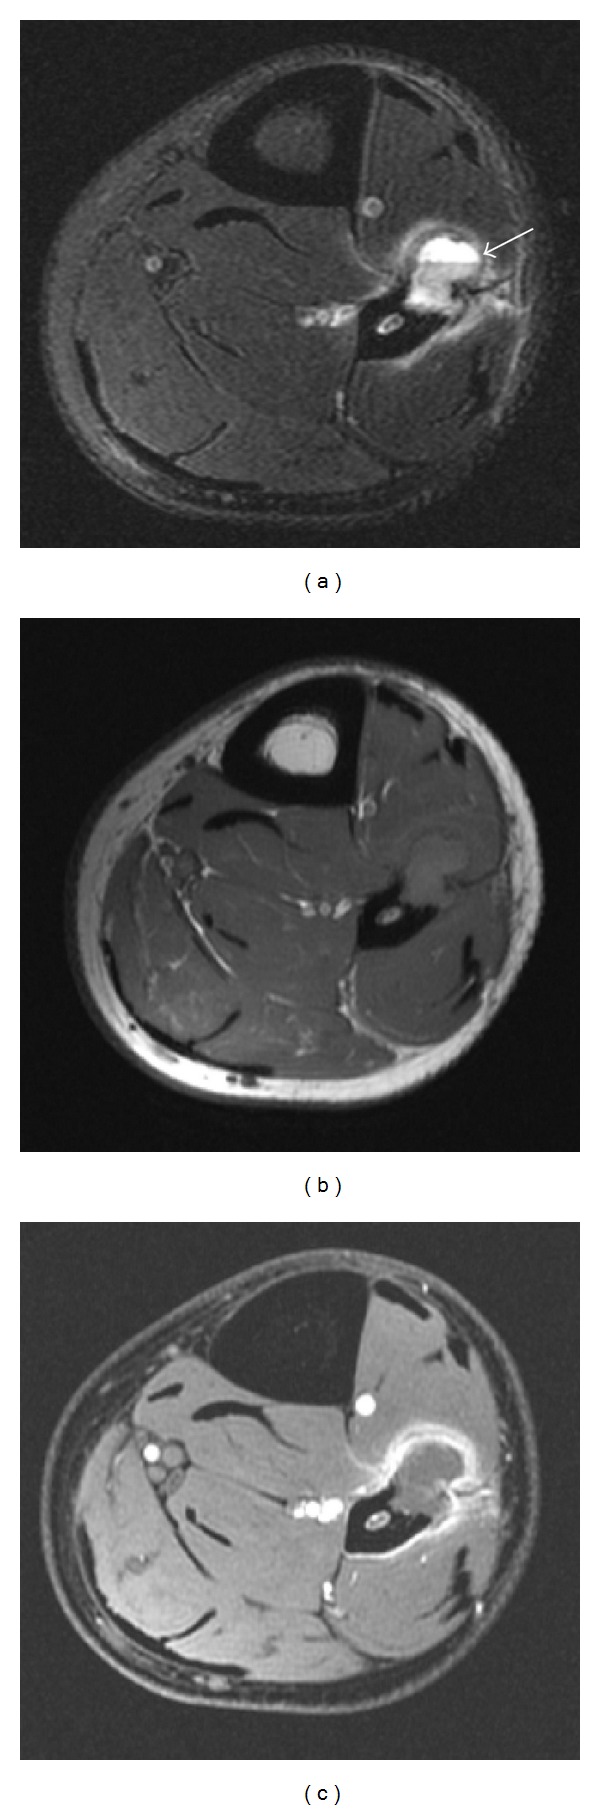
MRI of the distal fibula. (a) Axial T2-weighted MRI with fat saturation demonstrates a cortically based lesion within the distal fibula with a large fluid-fluid level (arrow). Peripheral increased T2 signal around the ABC is consistent with inflammatory changes. (b) Axial T1-weighted MRI shows the lesion is cortically based and has intermediate T1 signal within the mass. (c) Axial spoiled gradient with fat saturation following gadolinium shows peripheral enhancement only corresponding to the increased vascularity seen on color Doppler evaluation (Figure 1(c)).
